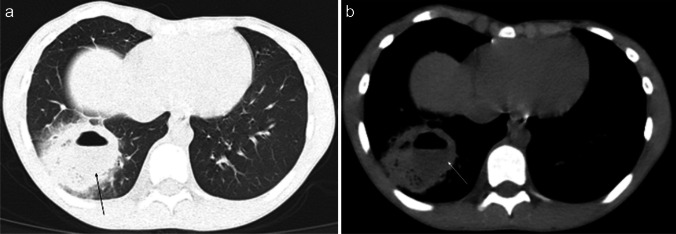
Axial non-contrast-enhanced computed tomography images in a 14-year-old boy with *Streptococcus anginosus* infection who presented with fever, cough, and right-sided chest pain. a Lung window image shows a round lesion with a thick wall, surrounding ground-glass opacities and an internal air-fluid level consistent with a lung abscess (*arrow*). b Mediastinal window image better demonstrates the air-fluid level within the lung abscess (*arrow*)
